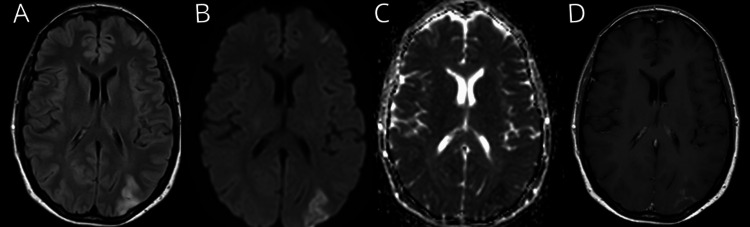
3T brain MRI, five days after symptoms onset. (A) FLAIR sequence shows bilateral parieto-occipital hyperintensities, predominantly in the left hemisphere.
